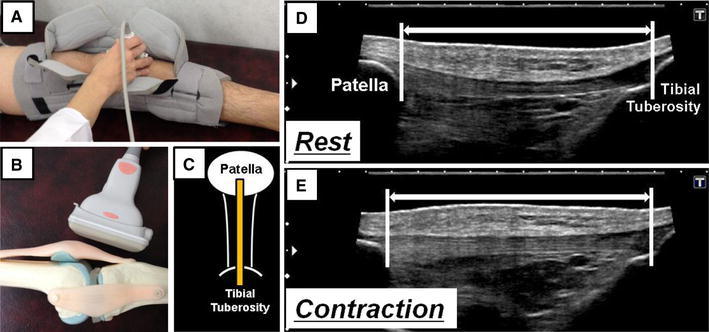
Ultrasound examination of the patellar mobility in the superior–inferior direction during isometric knee extension exercise. a The participants were evaluated in a supine position with the knee immobilized with a semi-flexed brace. b An ultrasound probe was fitted onto the skin overlying the patellar tendon in the sagittal plane using water bag kit. c The probe was positioned so that the caudal pole of the patella and the tibia tuberosity were visible within the viewing field. d, e Ultrasound images of the patella, patellar tendon, and tibial tuberosity at rest and during isometric knee extension contraction. The patellar mobility in the superior–inferior direction was measured as the change in distance between manually marked points of the deep insertion to the caudal pole of the patella and tibia tuberosity
